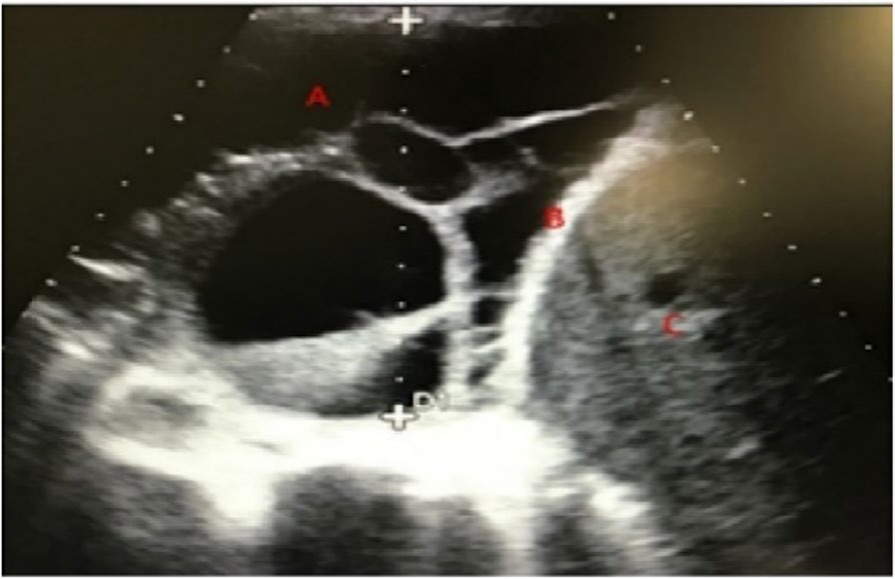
Displaying a transthoracic ultrasonographic image demonstrating a septated pleural effusion
